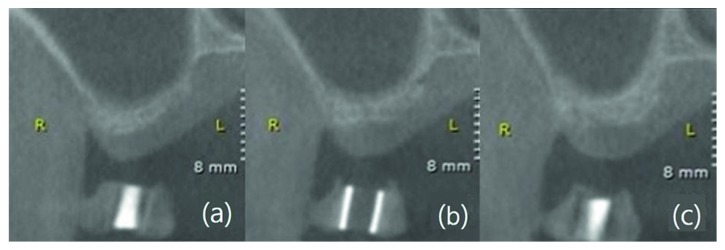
Residual bone height measurement method. Average value derived from (a) mesial, (b) central, and (c) distal aspects of the stent on computed tomography coronal view.
